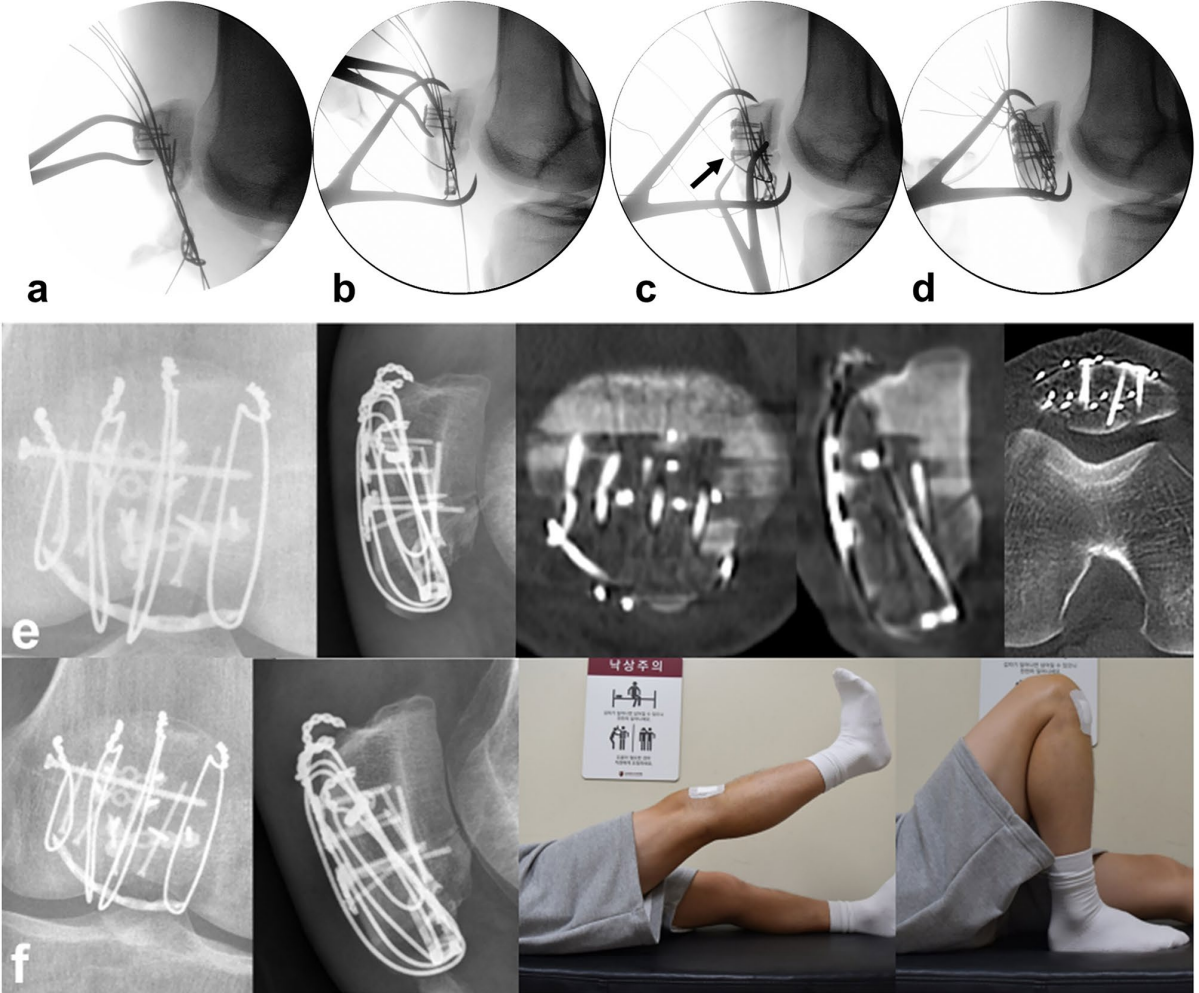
(a) Placement of rim plate and engagement of wires. (b) Reduction of proximal and distal fragments using reduction clamp. (c) An additional anterior cortical plate fixation for anterior cortical split fragment. (d) Tightening of vertical wires. (e) Postoperative radiographs show the rim-plate-augmented separate vertical wiring with supplementary fixation using two anterior cortical plates and embedded screws. All identified fragments were anatomically stabilized. (f) After 6 months, radiologic bony union was achieved and range of motion was fully recovered without extension lag.
